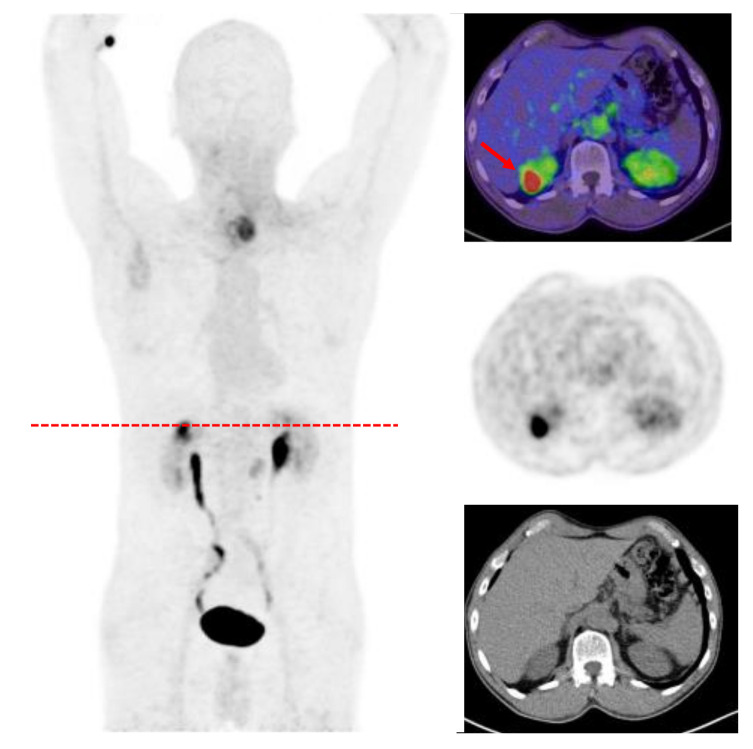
A 57-year-old patient underwent FAPI-PET/CT due to hypopharyngeal cancer and presented an incidental finding of an angiomyolipoma in the upper pol of the right kidney with a SUVmax of 15.9 as well as an accumulation located left paratracheal suspicious for neoplastic tissue (SUVmax 11.5).
